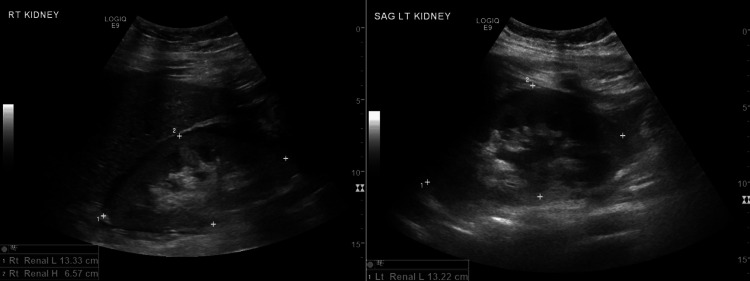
Renal ultrasound showing diffuse increased echogenicity of the renal cortices bilaterally which is consistent with medical renal disease, left mild hydronephrosis, and edema/fluid surrounding both kidneys RT: right, LT: left
